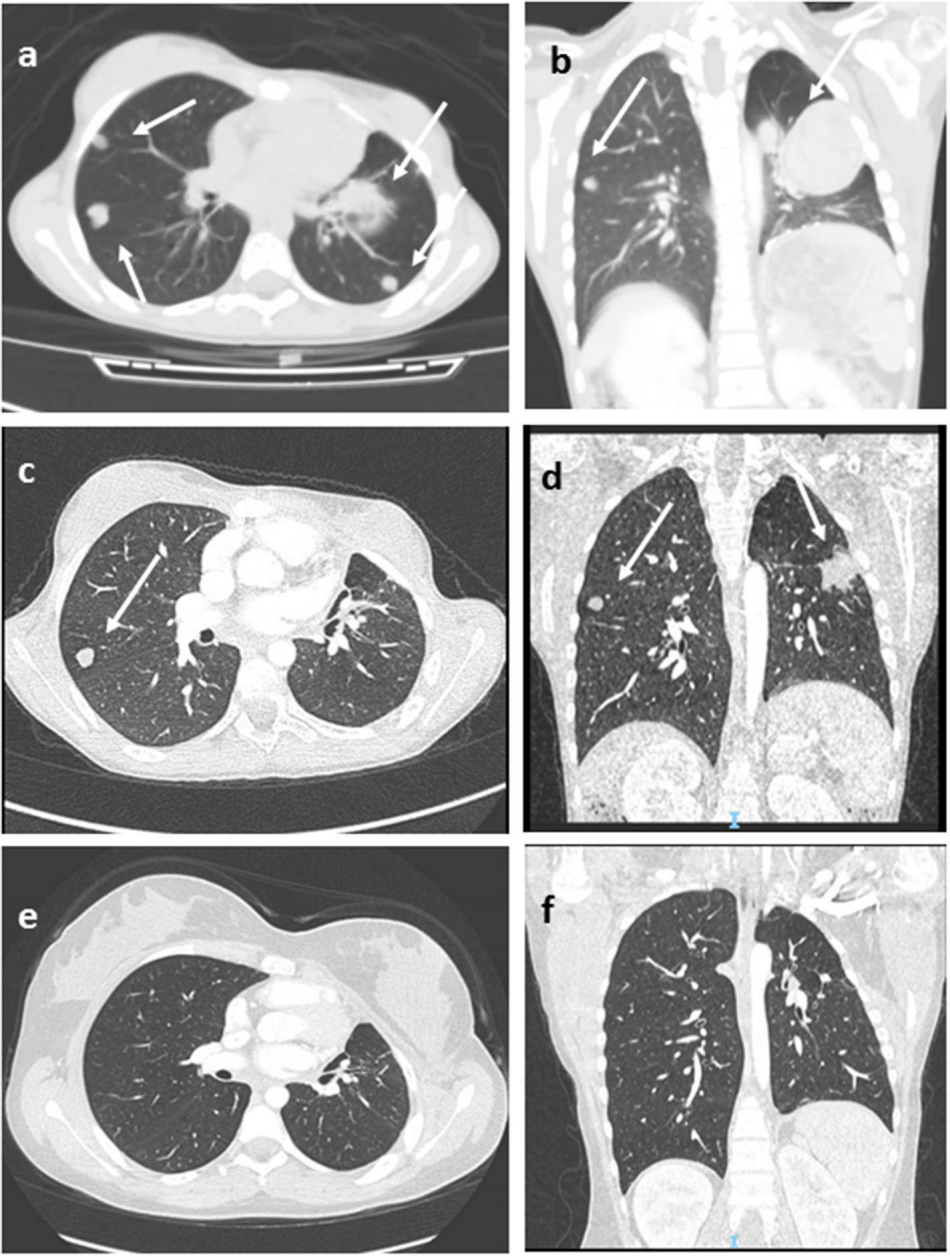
Lung Computed tomography (CT) images (patient #11) showing multiple metastatic lesions (arrows), before (a,b), after 3 months of pembrolizumab therapy (c,d), and at last follow-up (e,f) in complete disease remission. Axial (a,c,e), coronal (b,d,f).
